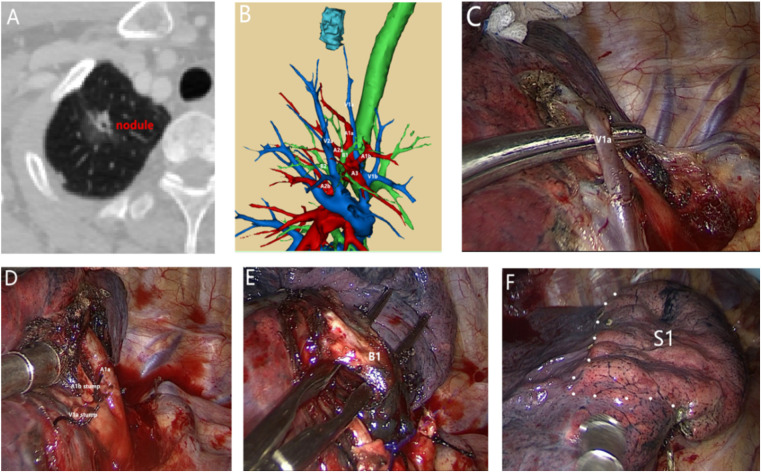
A 54-year-old male patient with nodules in the upper lobe of the right lung and a history of diabetes underwent right upper lobe apical segment resection. Postoperative pathology revealed invasive adenocarcinoma. (A) Preoperative computed tomography, right upper lobe apical nodules, 1.7 cm in diameter. (B) Preoperative 3D bronchovascular imaging of the right upper lobe apex segment. (C) Separation of V1a. (D) Separation and detachment of A1a and A1b. (E) Separation of B1. (F) S1 intersegment plane.
